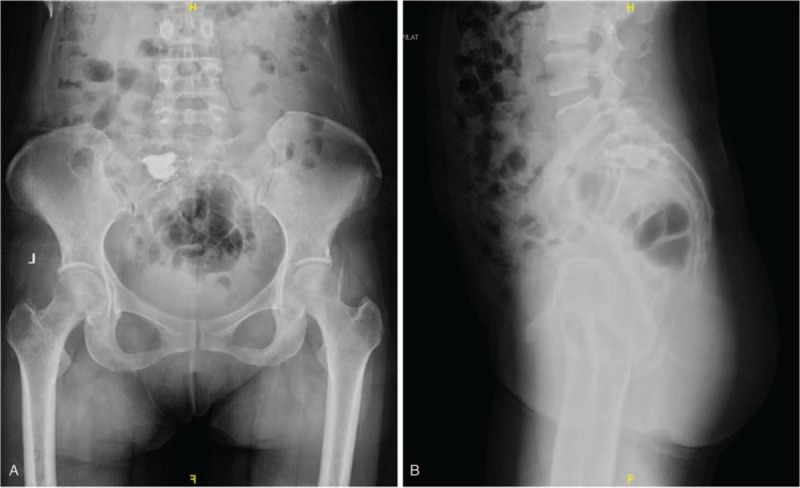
(A) Posteroanterior (PA) x-ray image of the sacrum obtained postoperatively. (B) Lateral x-ray image of the sacral spine obtained postoperatively.
